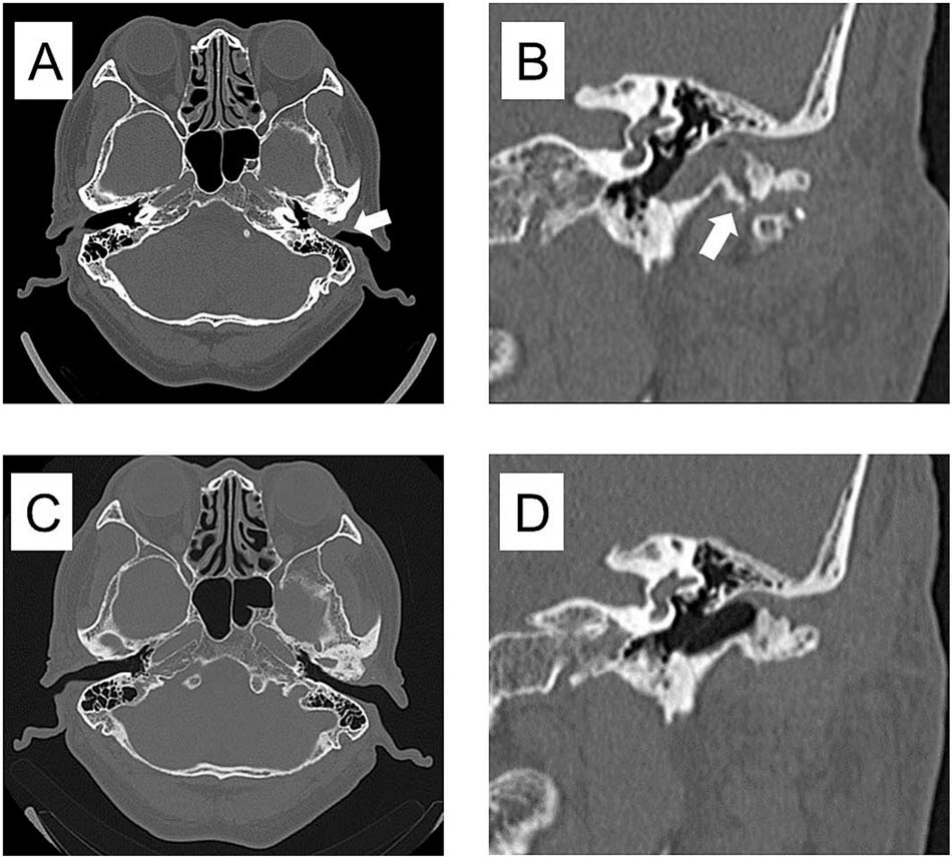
CT imaging findings when the mass was enlarged and the ear canal was obstructed and 3 years after surgery. (A) Axial section CT shows occlusion of the left external auditory canal. (B) Coronal section CT shows a bone defect from the temporomandibular joint to the external auditory canal. (C) Axial section of postoperative 3 years CT shows resolution of the mass in the external auditory canal. (D) Coronal section of postoperative 3 years CT shows restoration of bone defects from the TMJ to the external auditory canal.
